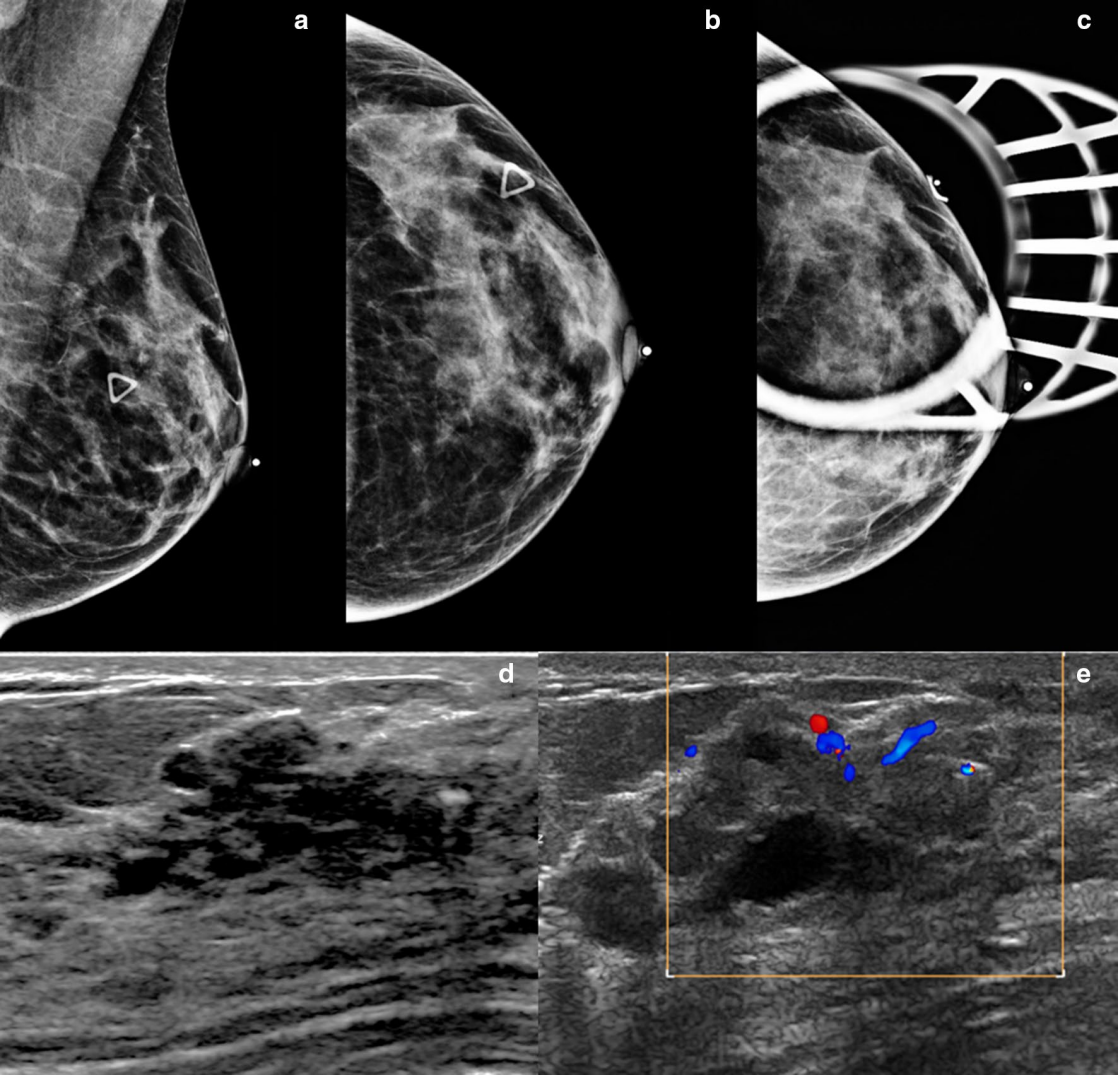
Sclerosing adenosis. A 35-year-old woman presented with a palpable breast mass. Mediolateral, craniocaudal, and spot tangential mammographic views do not reveal an abnormality (a–c, respectively). Grayscale and color Doppler ultrasound images (d, e) reveal a vascular, mixed cystic, and solid mass correlating with the area of palpable abnormality. Core needle biopsy showed sclerosing adenosis in a background of dense fibrosis without atypia or carcinoma. A 6-month follow-up ultrasound was rcommended and demonstrated stability
